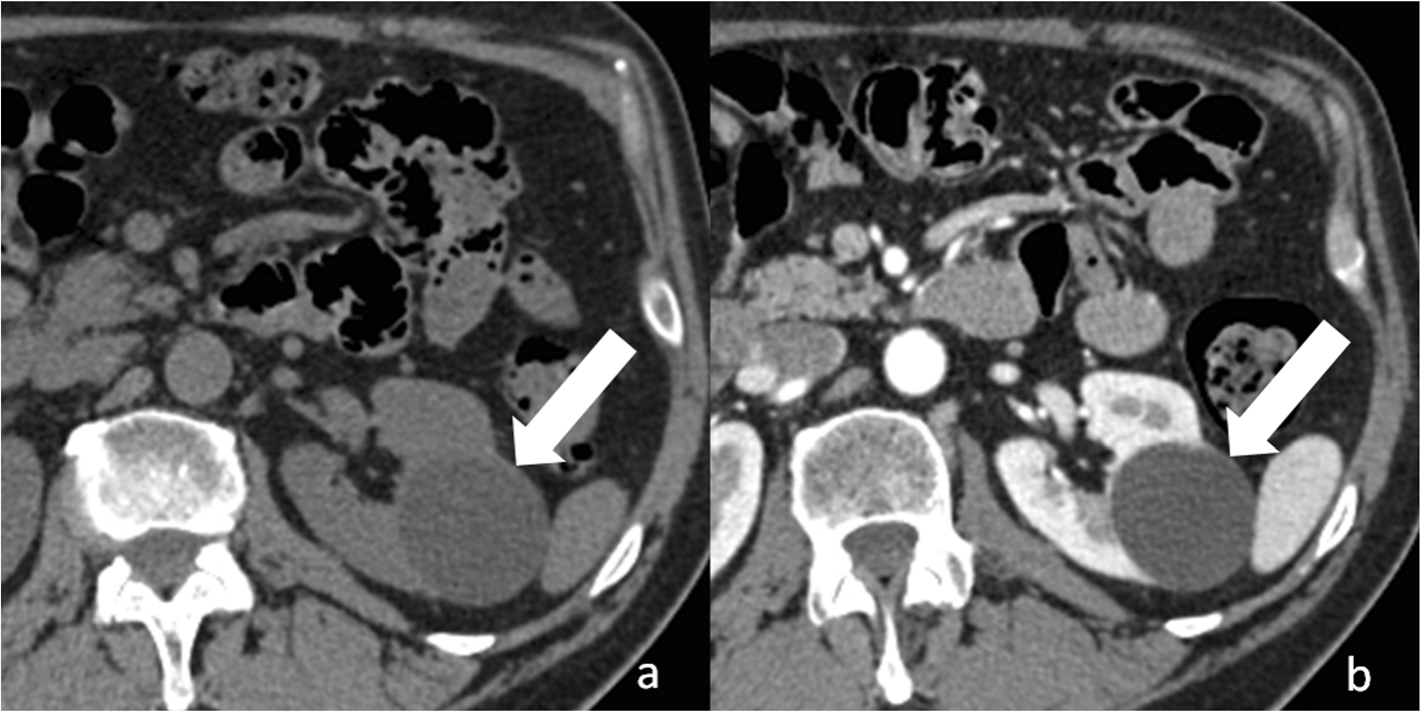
Bosniak category I renal cyst. Axial non-enhanced (a) and contrast-enhanced (b) CT images shows a cyst (arrow) with a thin and non-enhancing wall
